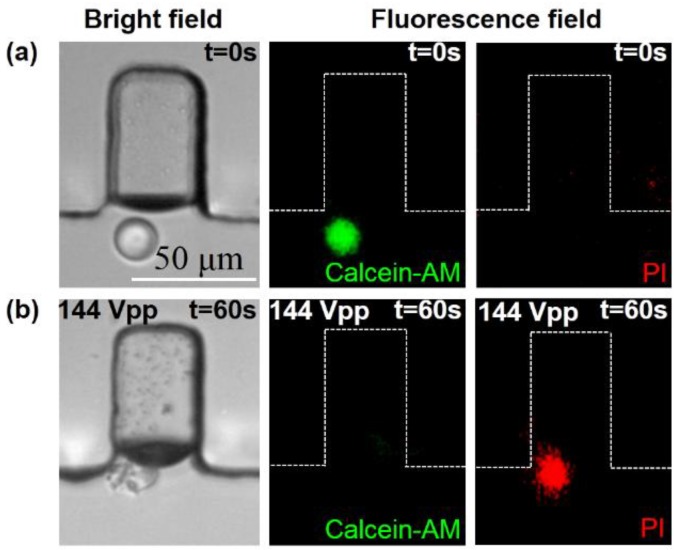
MCF-7 cell lysis. (a) The image series shows a single cell in the vicinity of the air bubble before the application of the ultrasound sonication. As the MCF-7 cell membrane is intact, the green fluorescence generated by the living cells is observed. (b) After the application of the ultrasound irradiation, the MCF-7 cell is broken into pieces, and only the red fluorescence of the PI is observed.
